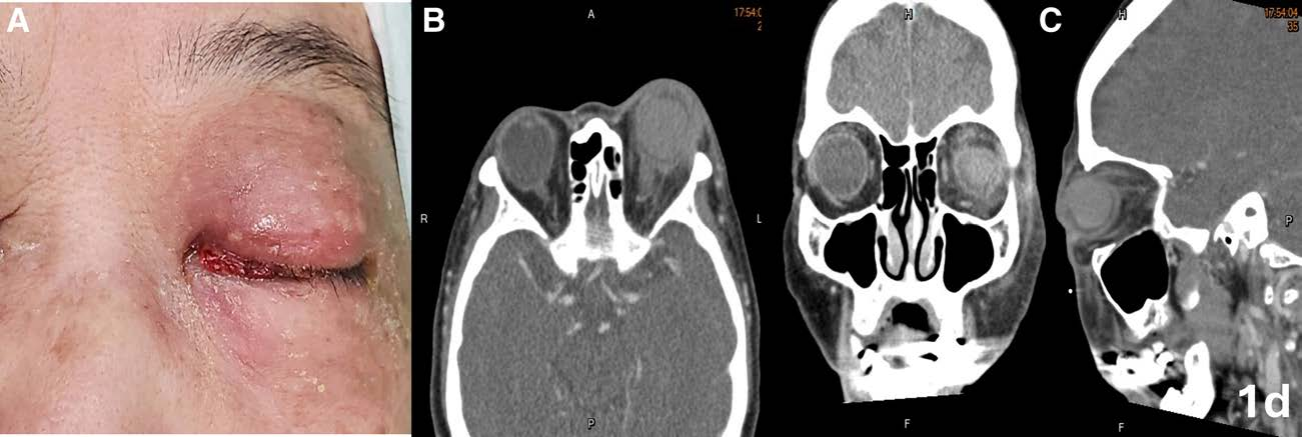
Initial presentation: (A) Severe eyelid swelling and firmness, preventing the eye from opening. (B–D) Axial, coronal, and sagittal CT scans show significant proptosis, hyperdensity in the vitreous and intraorbital fat stranding.
